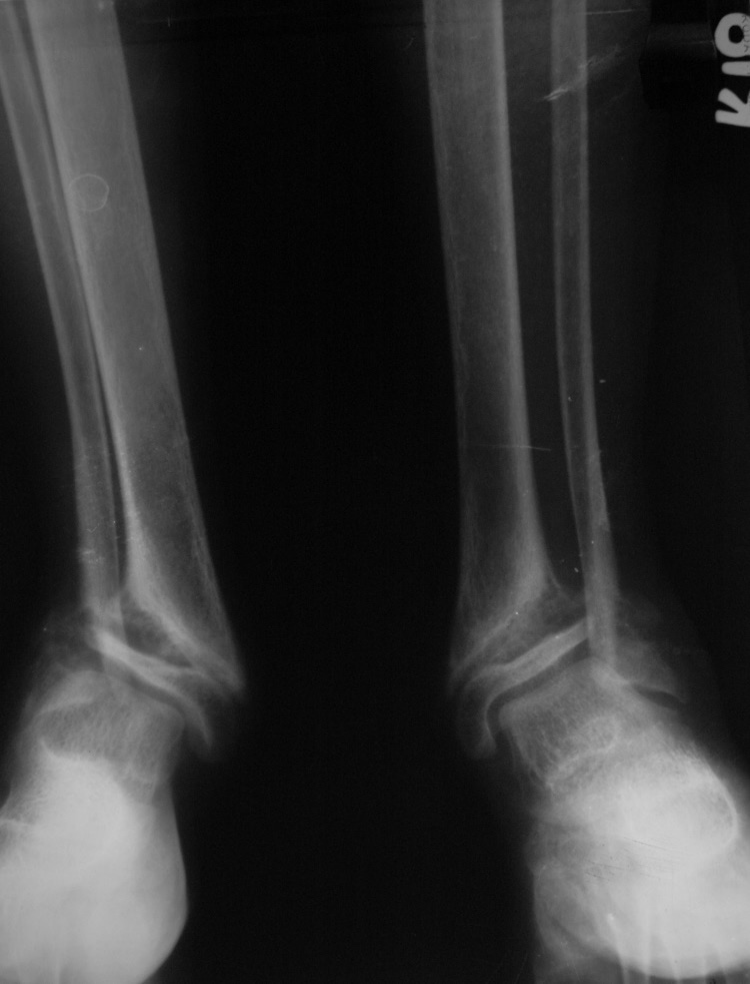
Radiograph of the ankle joints showing widening of the lower end of tibia, splaying and fraying of the metaphyses along with eversion deformity of the ankle joints
